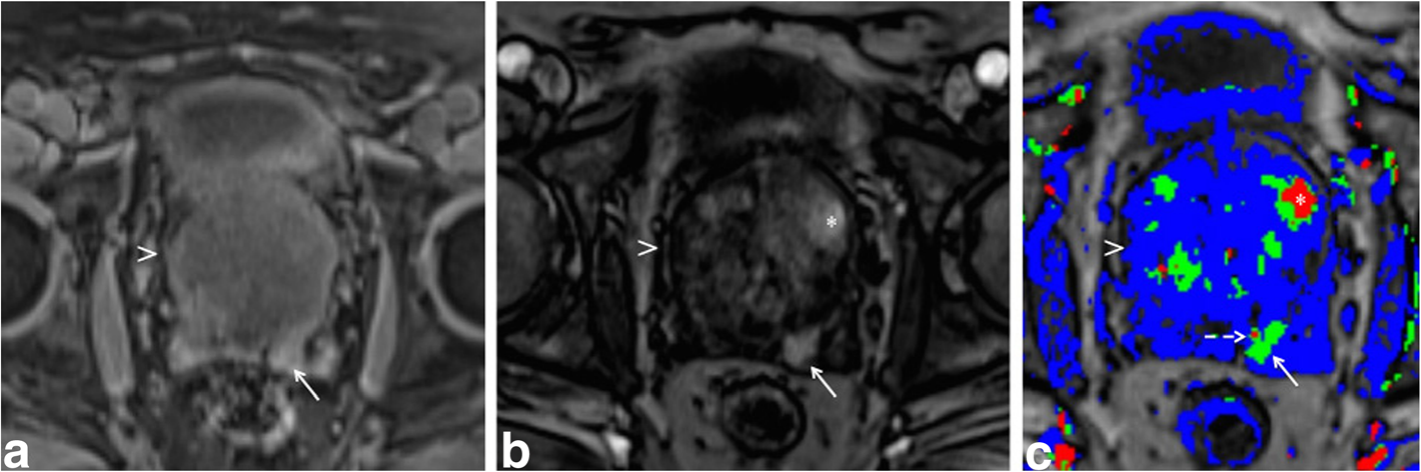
a-b Pre- and post-contrast fat-saturated T1-weighted (T1W) imaging of the prostate gland (*arrowhead*) and left seminal vesicle (*arrow*) reveal that this lesion demonstrated abnormal perfusion on dynamic contrast enhancement (DCE). c Computer-aided detection (CAD) confirms abnormal perfusion pattern of the lesion in the left seminal vesicle, which demonstrates early enhancement with partial washout and colorimetric stratification of both indeterminate/suspicious (‘*green*’) (*dashed arrow*) and highly suspicious patterns (‘*red’*) (*solid arrow*). The hyperperfused lesion in the left central zone with highly suspicious enhancement on CAD (*asterisk*) demonstrated relative hypointensity on T2W imaging with thin, circumscribed hypointense periphery, and no diminished diffusivity, compatible with a hyperplastic (BPH) stromal nodule that was later confirmed as benign on MR imaging-TRUS fusion biopsy
